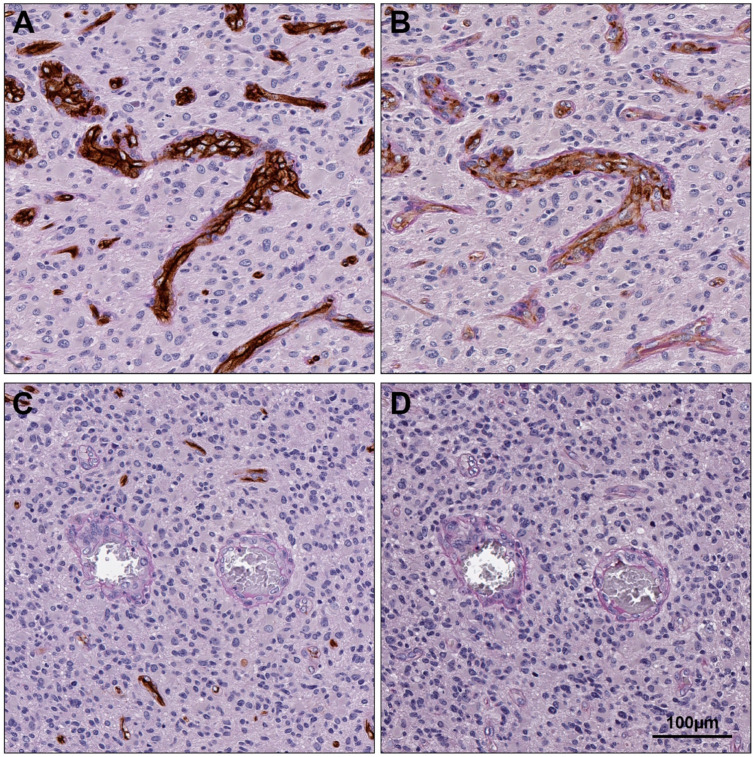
PSMA is expressed by endothelial vessels. Serial sections labelled by IHC for CD34 (A,C) and PSMA (B,D) demonstrate PSMA labelling in CD34+/PAS+ endothelial vessels, but not CD34−/PAS+ VM vessels. Scale bar = 100 µm.
